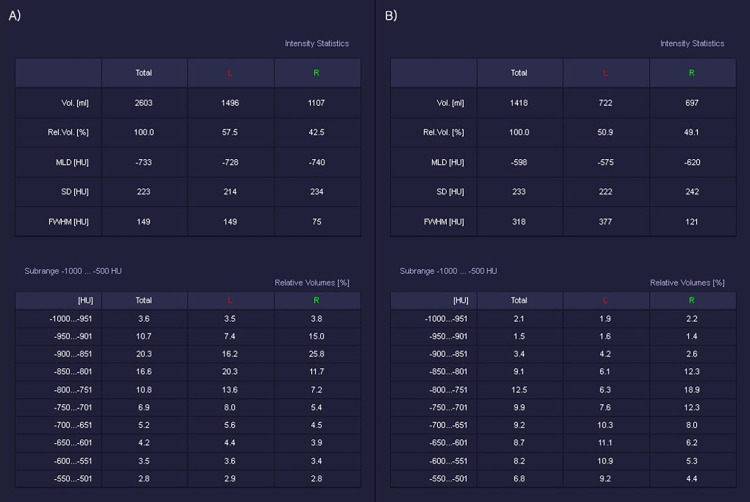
Example of densitometry parameter measurements using the 3D chest CT of a representative subject in the A) inspiratory and B) expiratory phases.
